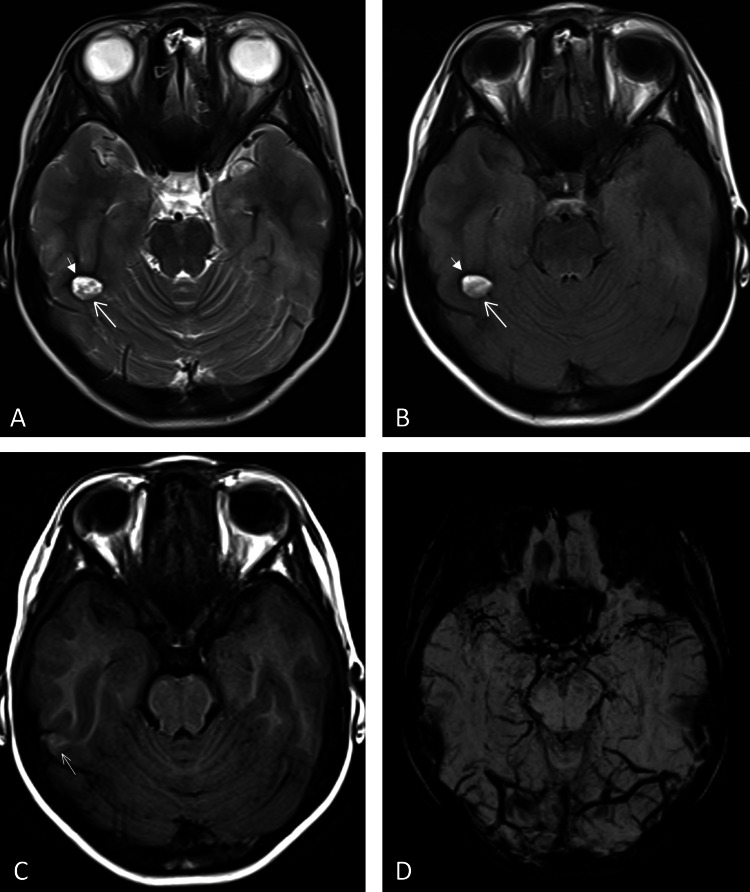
Characterization of the artifact in Case 3 (A) Axial T2 and (B) axial T2FLAIR sequences demonstrate an oval-shaped heterogeneous hyperintense lesion in the right temporal lobe with well-defined margins(arrows). A hypointense rim (short arrows) is visible along the anterior margin, while the posterior margin is absent. On (C) axial T1, a small patchy moderately hyperintense signal is observed with ill-defined margins, and it is slightly more lateral compared to its location on T2 and T2FLAIR sequences(arrow). On (D) SWI sequences, no abnormal signal is detected at this anatomical location. SWI: Susceptibility-weighted imaging, FLAIR: Fluid-attenuated inversion recovery.
